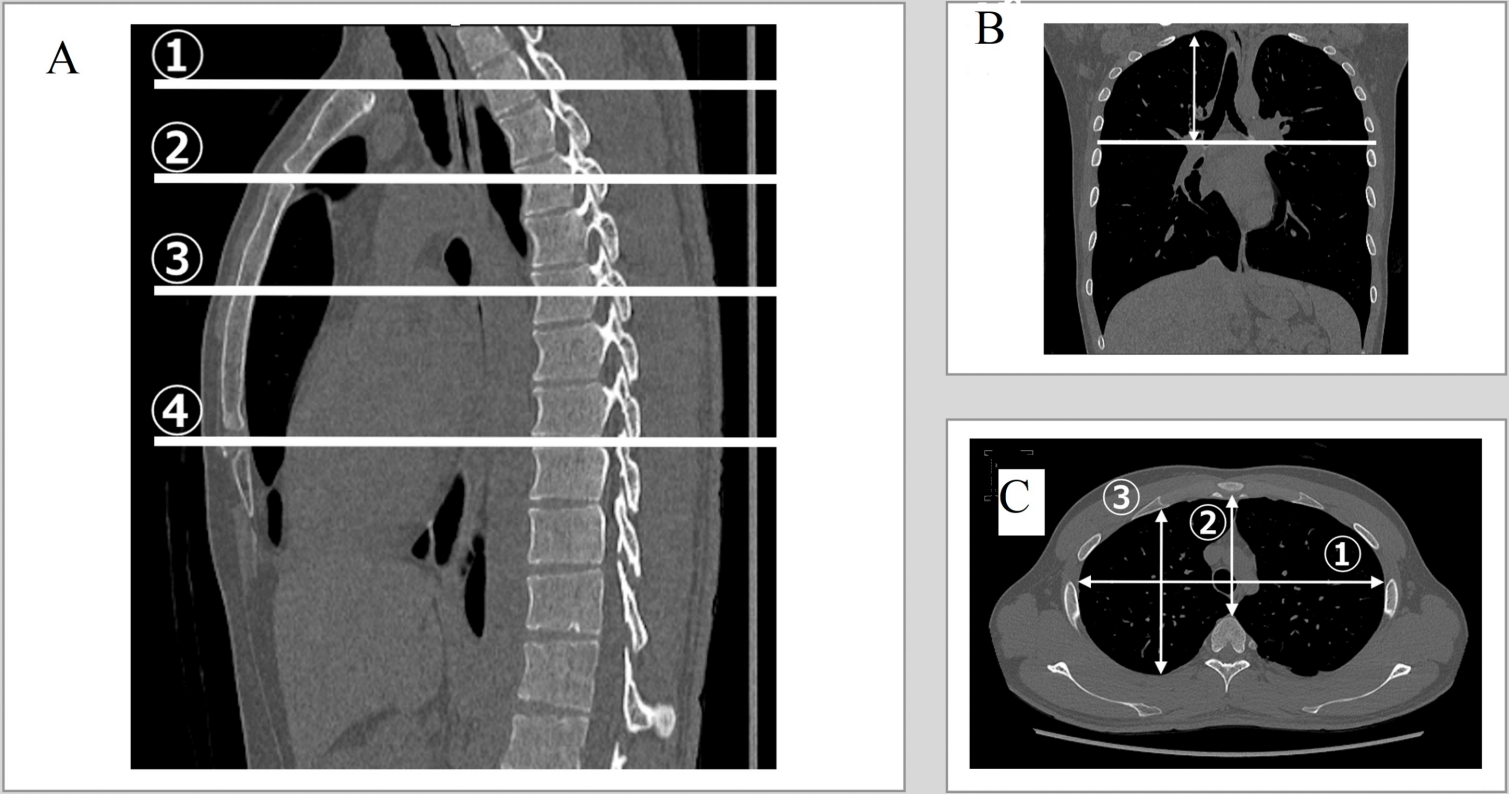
Thoracic measurement using CT (A) Sagittal section. Four heights were specified in the horizontal section. ① manubrium, ② sternal angle, ③ midpoint of sternal body, and ④ xiphi-sternal angle. (B) Horizontal section. Transverse diameter ①, sagittal diameter ②, and right and left anteroposterior diameters ③ were measured in horizontal section. The sagittal diameter was defined as the length from the back surface of the sternum to the anterior margin of the vertebral body. (C) Coronary section. The length from each of the four levels to the apex of the thorax was measured
